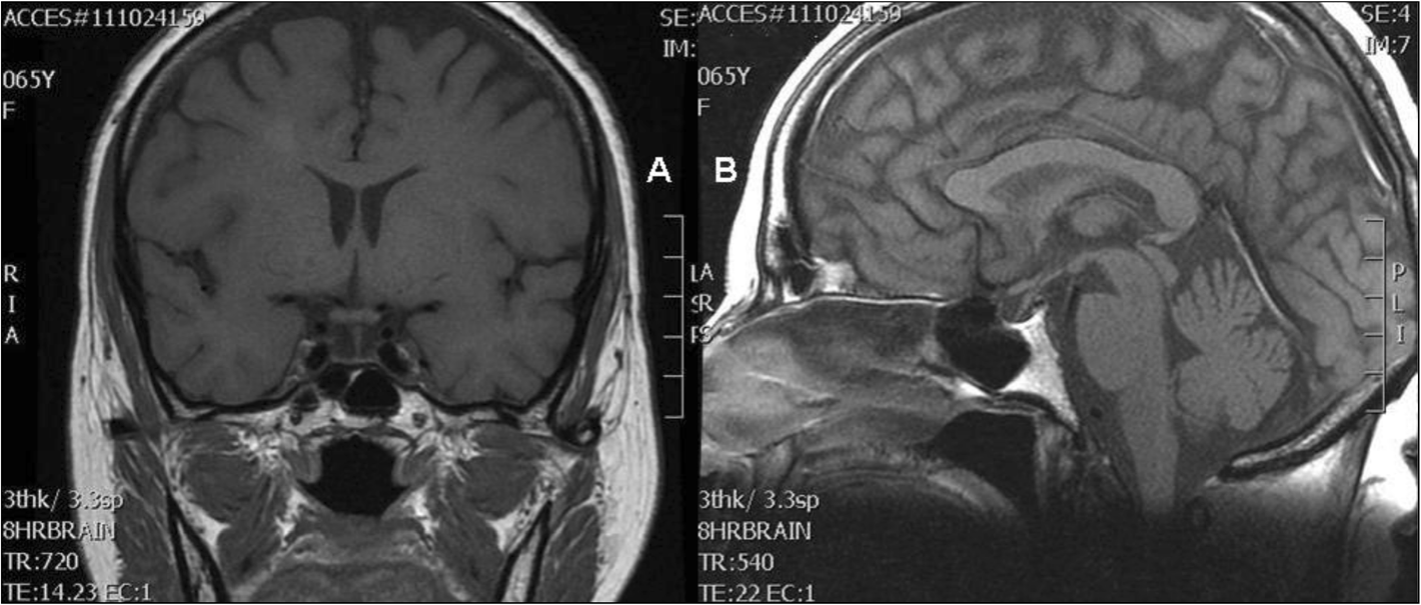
Coronal (A) and sagittal (B) T1-weighted magnetic resonance imaging. Images show marked diminution in the size of the pituitary gland. The pituitary fossa appears normal in size. The pituitary stalk is well seen and correctly oriented.
